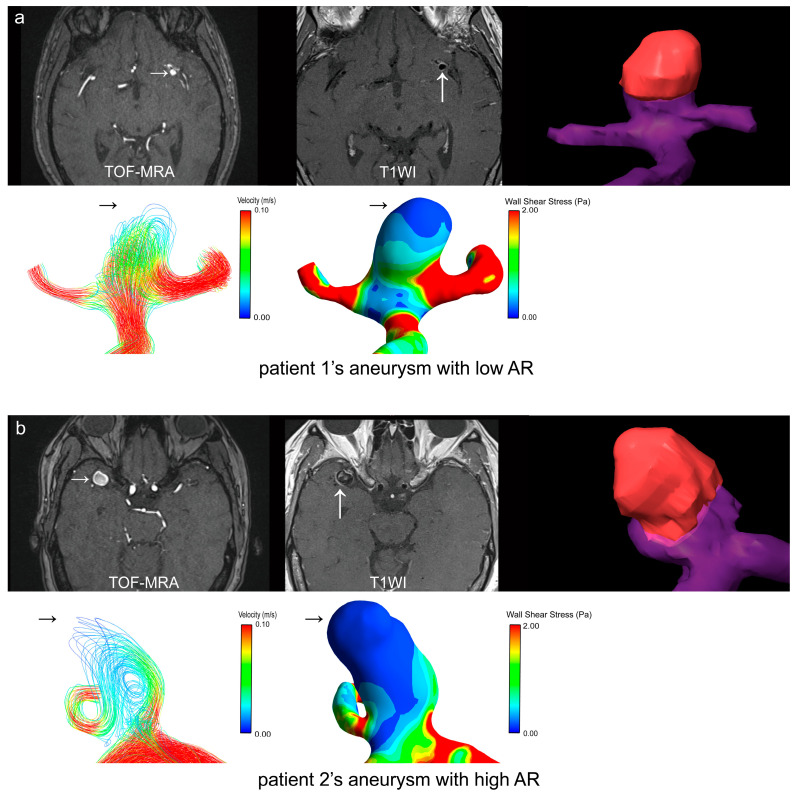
Images of the patients’ aneurysms with different ARs. The auto fusion of the TOF-MRA (small white arrow) and VW-MRI T1WI (large white arrow). The AWE area is highlighted in red and the aneurysm with parent artery is highlighted in purple. (a) The enhanced area (black arrow) of the aneurysm with the low AR of patient 1 has a lower proportion of low-WSS and low-velocity regions. (b) The enhanced area (black arrow) of the aneurysm with the high AR of patient 2 has a larger proportion of low-WSS and low-velocity regions.
